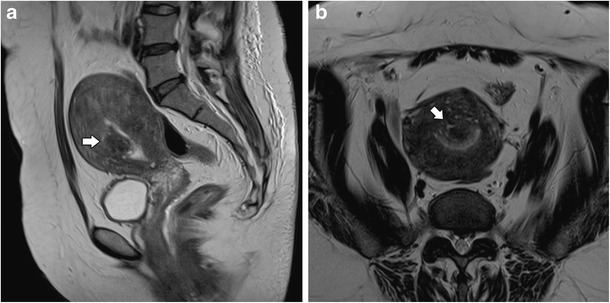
Polypoid adenomyoma: a Sagittal T2- and b Axial T2-weighted images; projection of junctional zone into the endometrial cavity with nodular morphology and ill-defined borders (white arrows)
